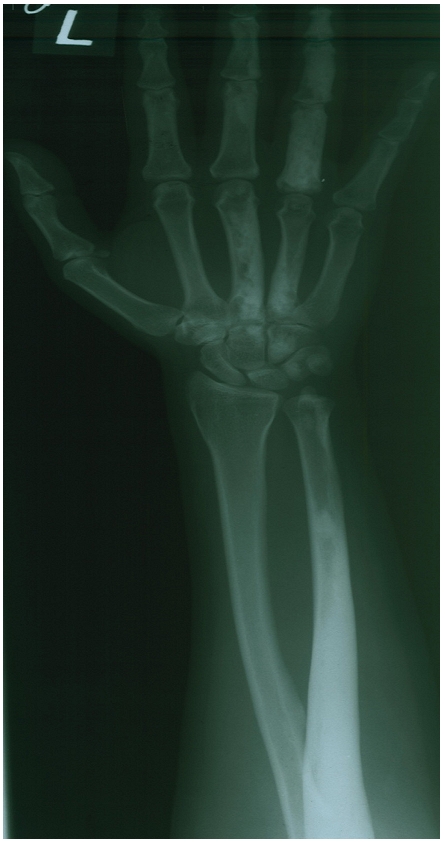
Plane radiographic images showing hyperostosis of the 3rd and 4th metacarpal and digits as well as ulna and distal humerus
